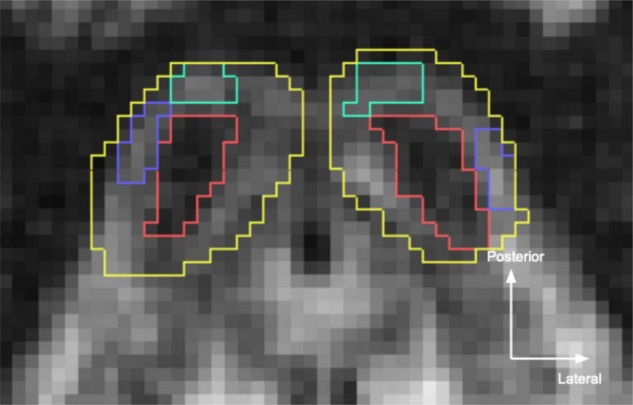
Inferior colliculus (IC) segmentations in the sub-millimeter *in vivo* dataset. Yellow, overall IC; red, central nucleus (ICc); turquoise, dorsal nucleus (ICd); blue, external nucleus (ICx). Segmentations were hand drawn on the 760 μm isotropic diffusion MRI quantitative anisotropy map.
